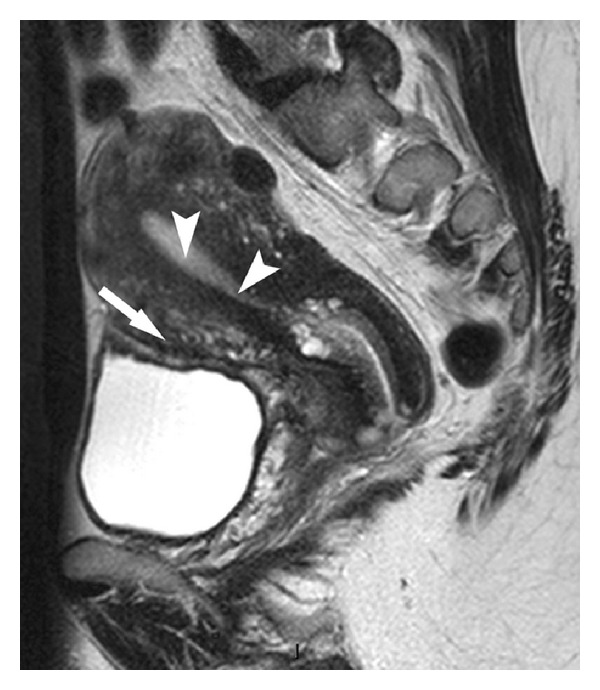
The T2 weighted postoperative pelvic MR image shows no residual myoma. Note the complete uterine cicatrization (arrow) and the regular endometrial cavity with no defect visible (arrowheads).
